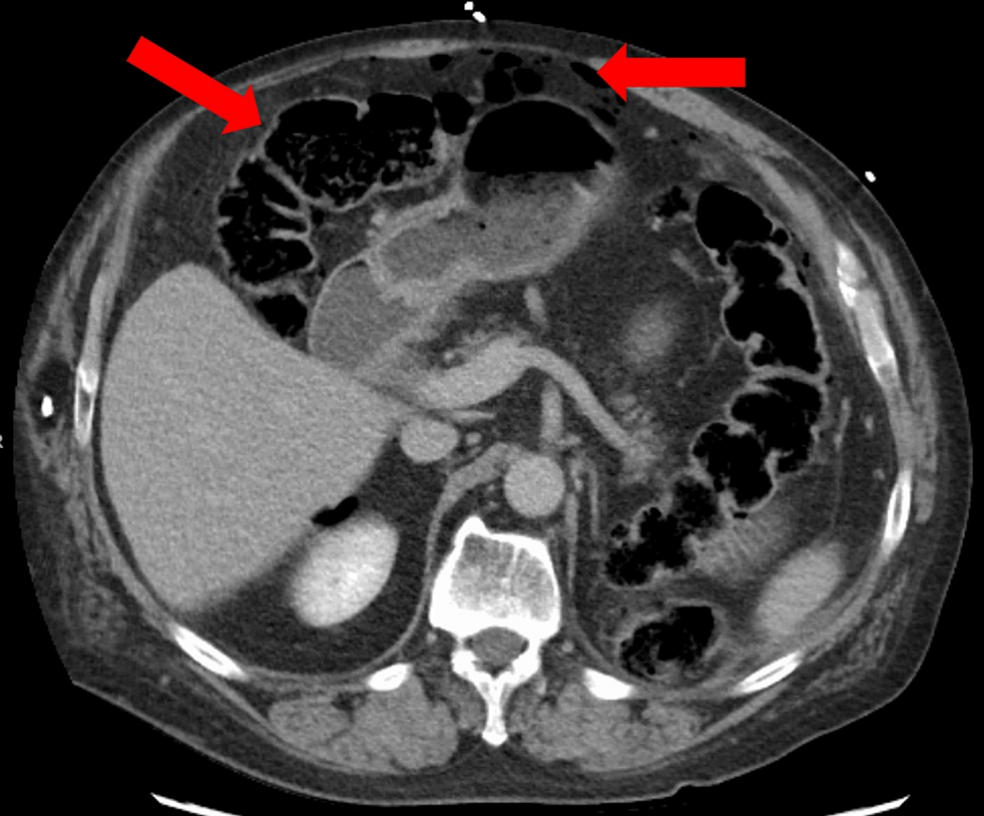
CT of the abdomen and pelvis showing diffuse pneumoperitoneum consistent with a bowel perforation and dilated loops of small bowel.
